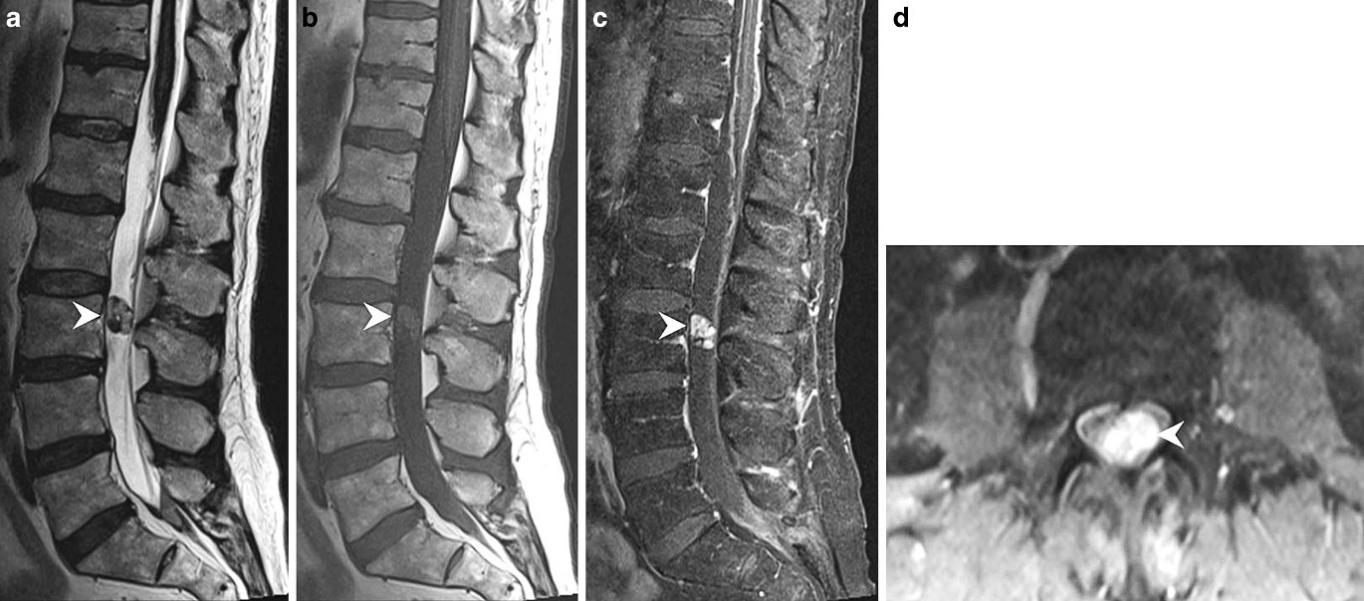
On sagittal T2-weighted images (a) a heterogeneous lesion (*arrowhead*) at the L3 level can be appreciated. The lesion shows slightly elevated signal intensities on nonenhanced sagittal T1 weighted images (b, *arrowhead*) when compared to cerebrospinal fluid. After administration of gadolinium the lesion shows distinct contrast-enhancement on sagittal (c), and axial (d) T1-weighted images (*arrowhead*)
